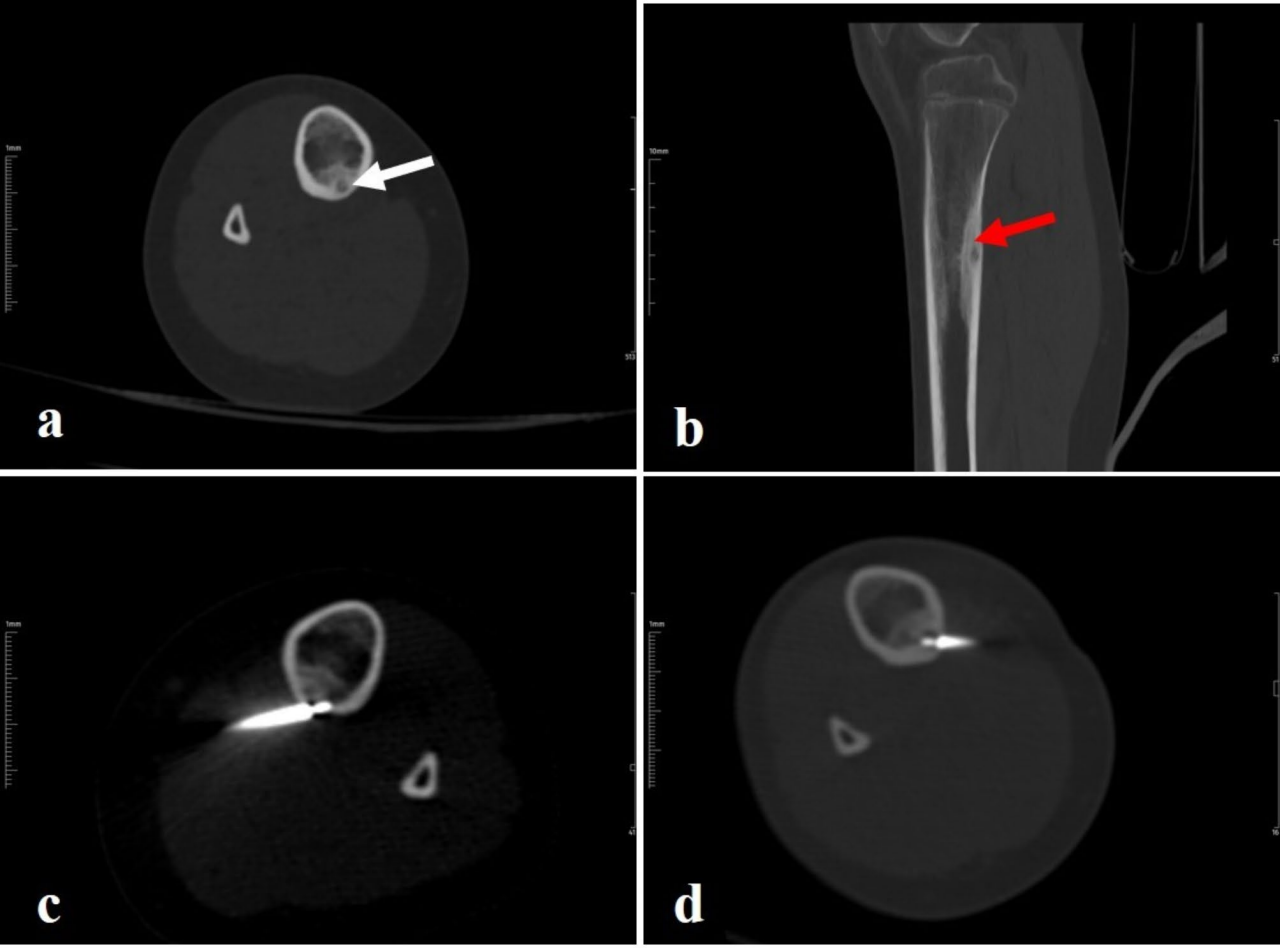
In an 9-year-old female patient recurrence is seen after the RFA. a.) on axial images, b.) on coronal images the lesion located in the cortex of the proximal tibia is seen on CT images. The lesion is peripherally sclerotic and centrally hypodense. the nidus (white arrow) diameter was 4.7 mm, and the periosteal reaction (red arrow) thickness was 4.5 mm, c.) the image is shown first RFA, d.) the image is shown second RFA, after the first ablation. The pain score was 9 before the first ablation, the pain score after RFA was 3 points at the 1st week and 4 points at the 3rd month. The patient was using NSAIDs 7 times a week before the first procedure, she started taking NSAIDs 4 times a week after the first ablation. Our patient was considered to have relapse because the patients’ pain and NSAID use did not improve effectively. A second RFA was performed 6 months after the first ablation. Before the second RFA, the patient had a pain score of 7 and was taking NSAIDs 4 times a week. After the second RFA, the pain score decreased to 0 points in the 1st week and 3rd month. NSAID use was also 0
